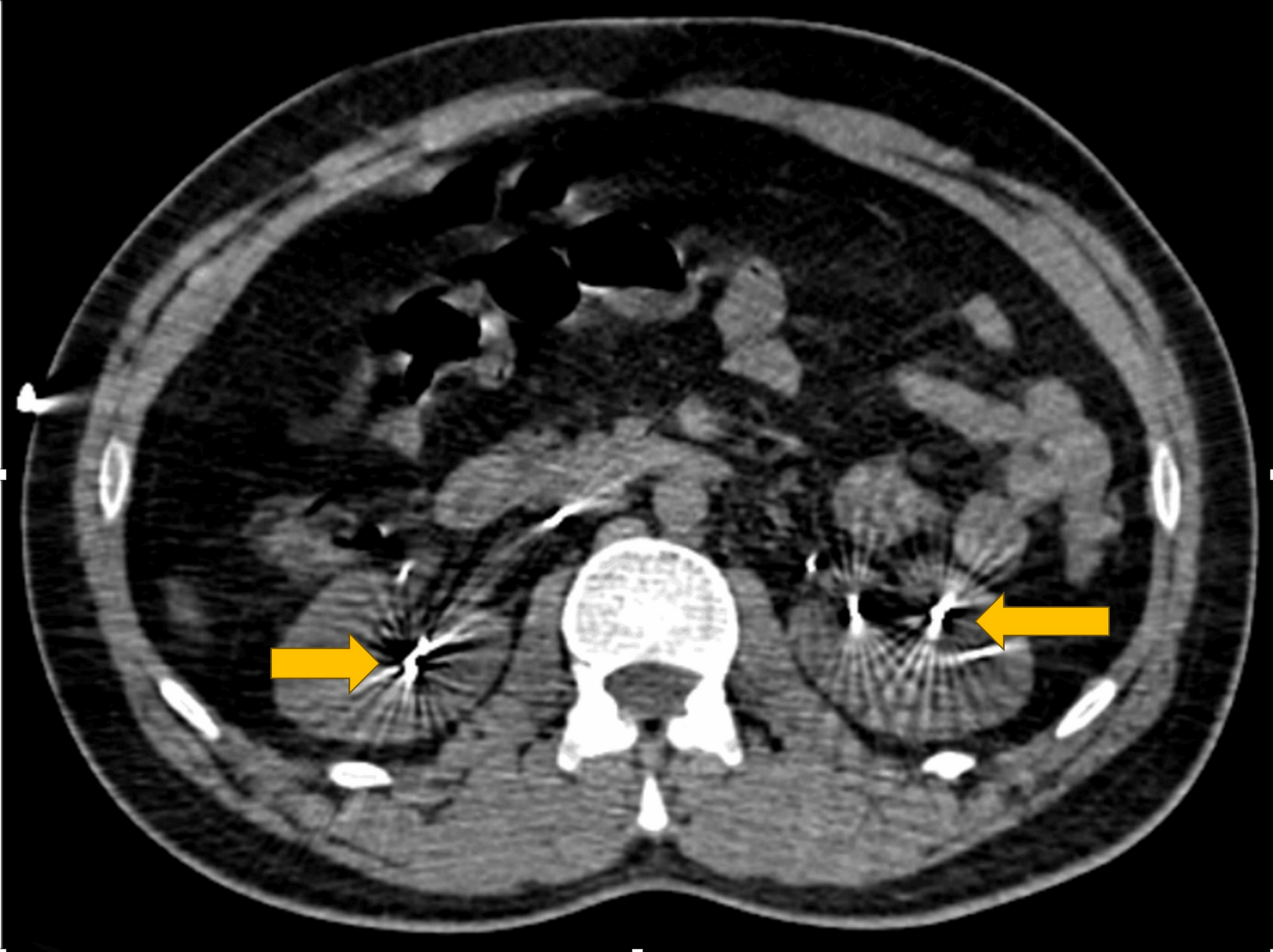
CT scan of abdomen and pelvis axial section (soft tissue window) at the level of bilateral kidneys shows foci of high attenuation in bilateral kidneys (yellow arrows), suggesting excretion of mercury through kidneys.
